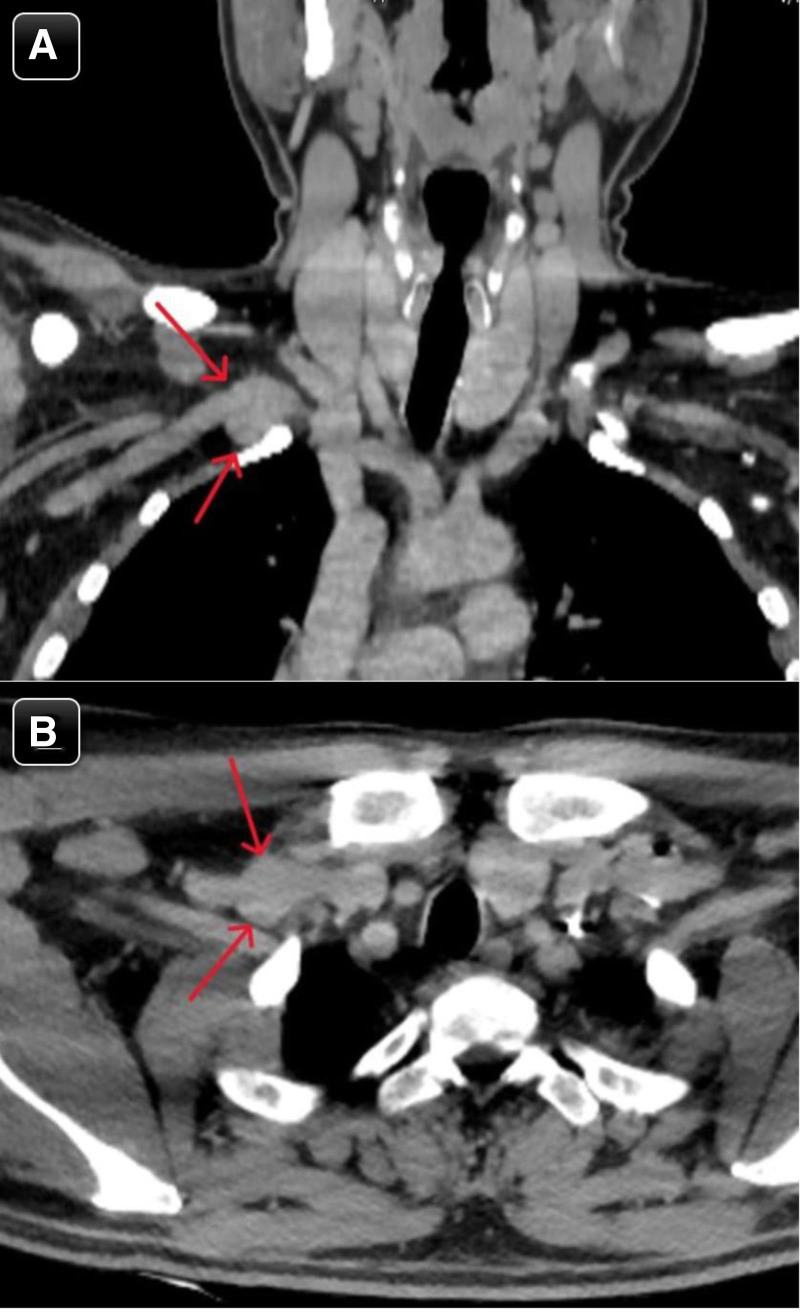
(A & B) Coronal and axial view of neck CT angiography showed aneurysmal fusiform dilation of the proximal right subclavian vein.
